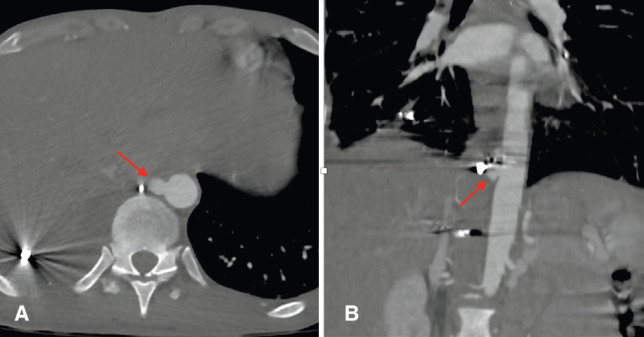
CT scan revealing a 9 mm x 9 mm x 6 mm aortic pseudoaneurysm with two metal shards near this site. Red arrow indicates the pseudoaneurysm: (A) Axial view; (B) Coronal view
